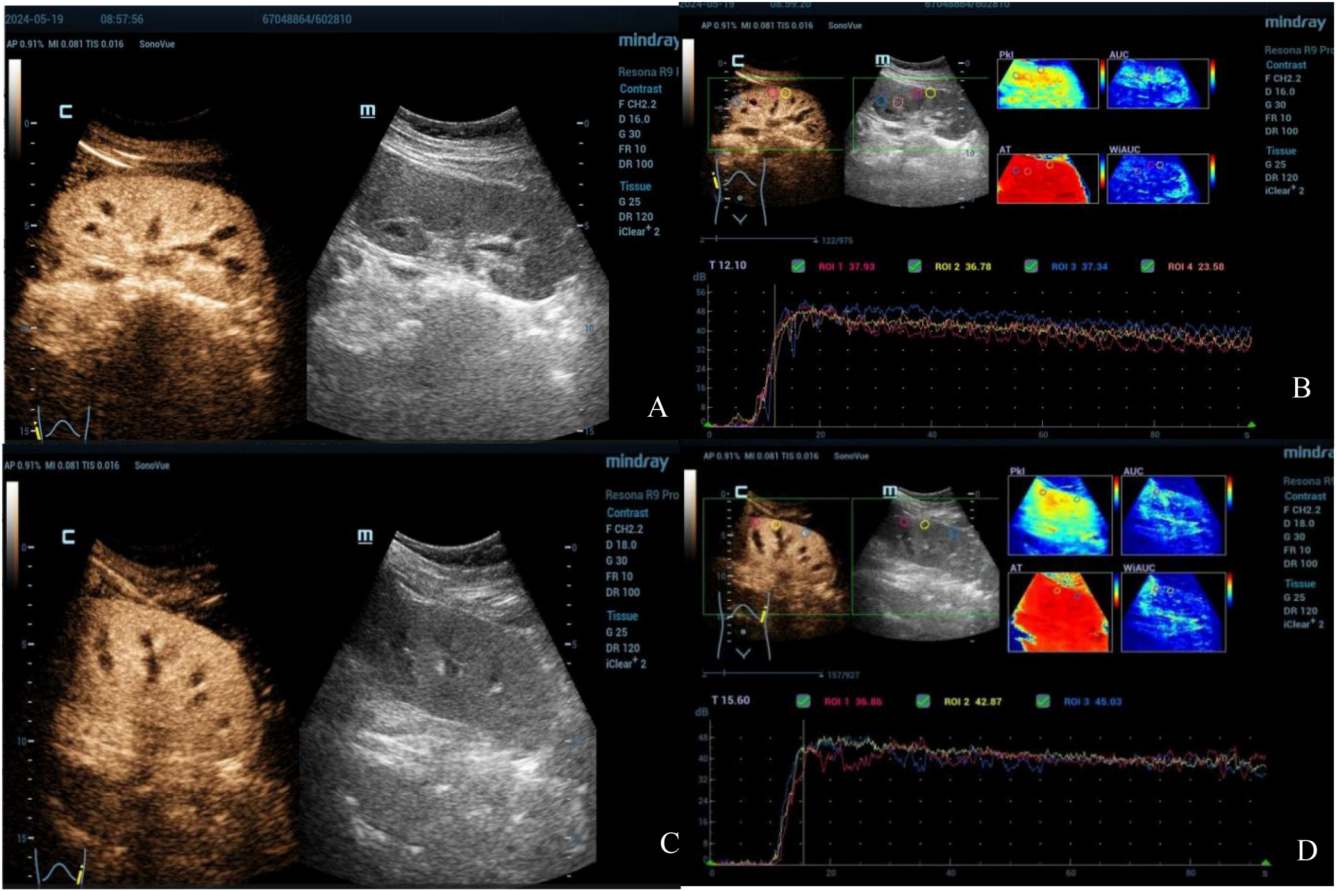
Contrast-enhanced ultrasound images of both kidneys in the second patient (Right: A,B; Left: C,D). The images reveal good cortical blood wash-in and perfusion but significantly delayed wash-out and excretion.
